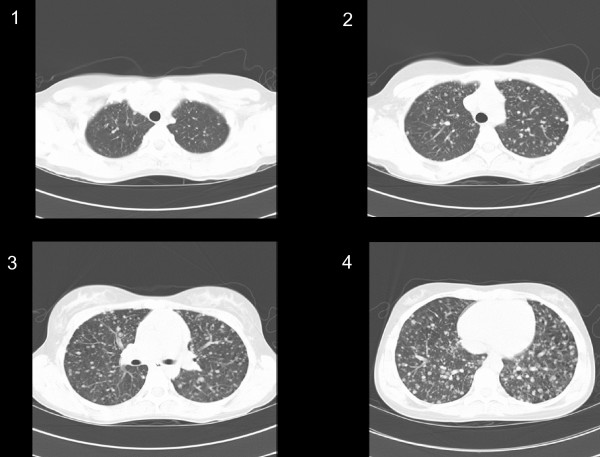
CT scan of the chest that cuts from the apices (1) to the lung bases (4) and shows tremendous tumor burden as well as a basilar prominence of metastases typical of papillary thyroid carcinoma lung metastases.
